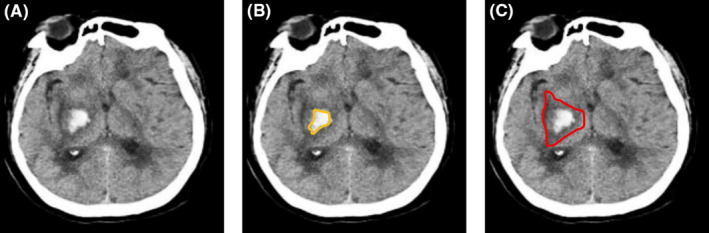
The measurement of PHE volumes on one representative CT image. (A) A representative CT image of ICH at right basal ganglia; (B) The hematoma area (hyperdense lesion, Hounsfield unit 40–100) was manually circled in yellow; (C) The total lesion area [PHE (the area in hypodensity surrounding hematoma, Hounsfield unit 5–33) plus hematoma] was manually outlined in red. The area of PHE on each slice was calculated by subtracting the hematoma area (yellow) from the total lesion area (red). Finally, the PHE volume = ∑ (PHE area in each slice) × the thickness of each slice. ICH, intracranial hemorrhage; PHE, perihematomal edema.
